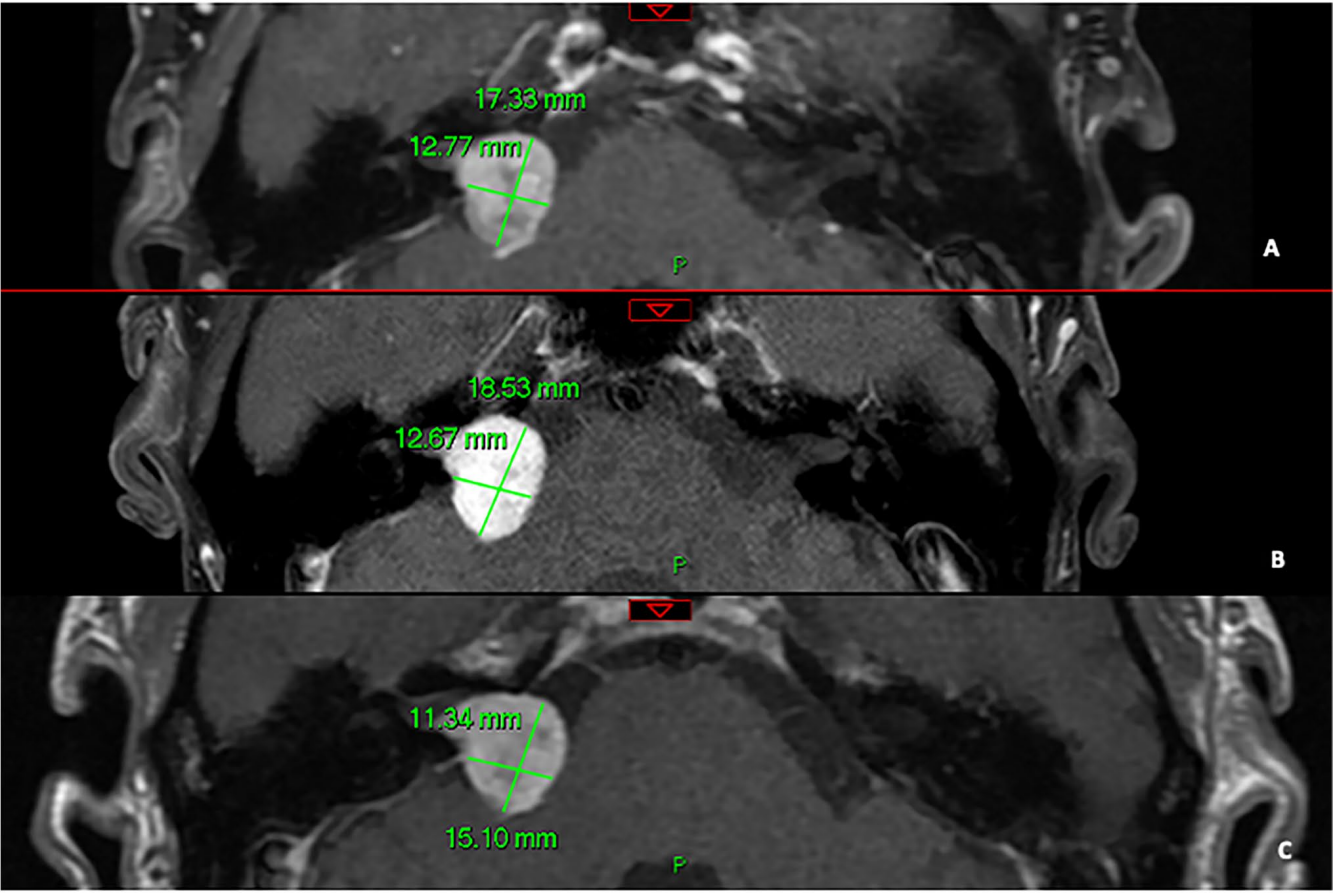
Post-SRS axial, T1-weighted post-gadolinium 1.5 T in a male patient with right VS (A) showing the increase in size 6 years post-treatment (B) and further stabilisation nearly 2 years later (C)
